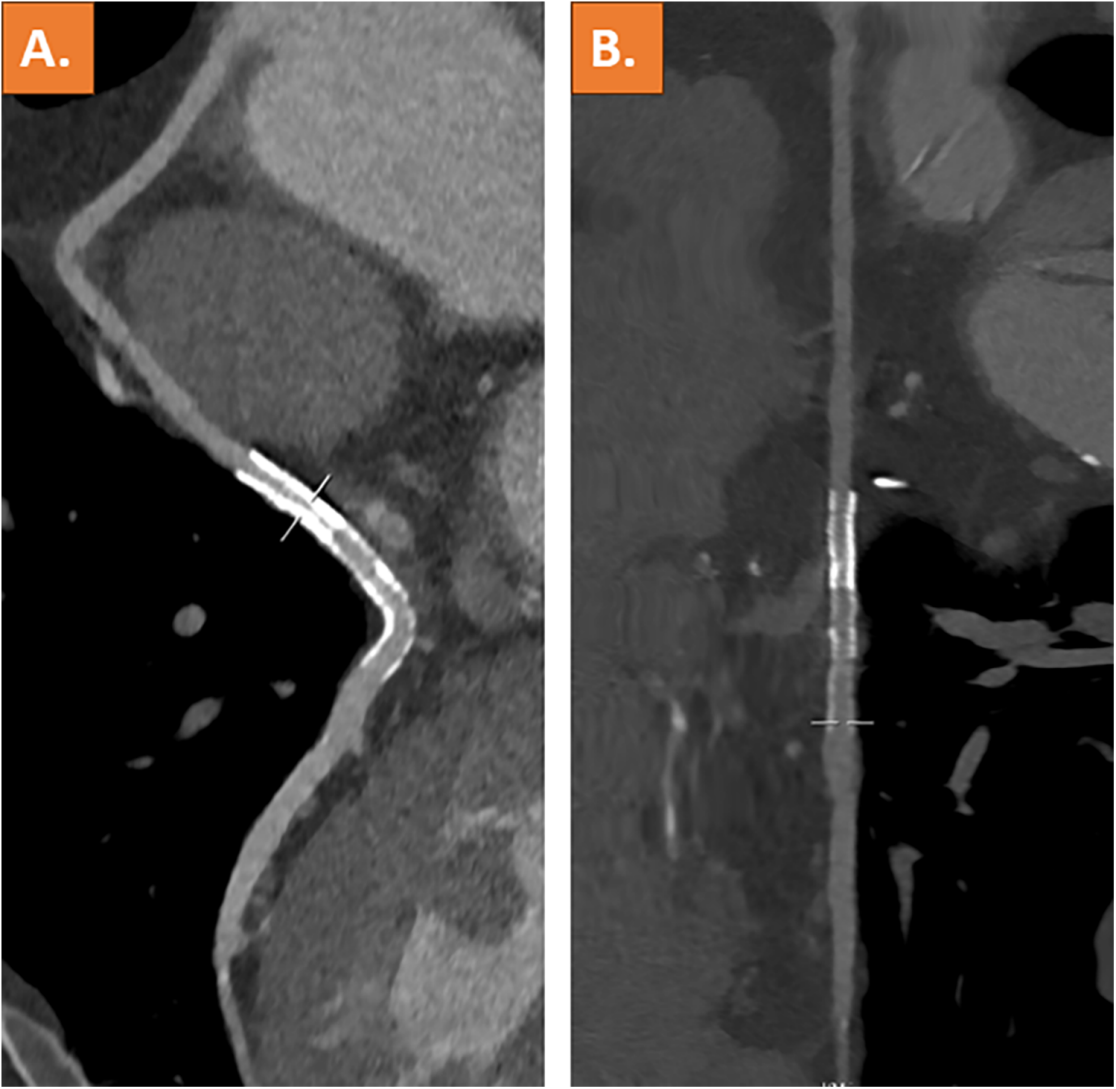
Coronary computed tomography angiography with curved multiplanar reformation (A) and stretched vessel view (B) showing a patent venous coronary artery bypass graft including stent lumen and complete resolution of the thrombus.
